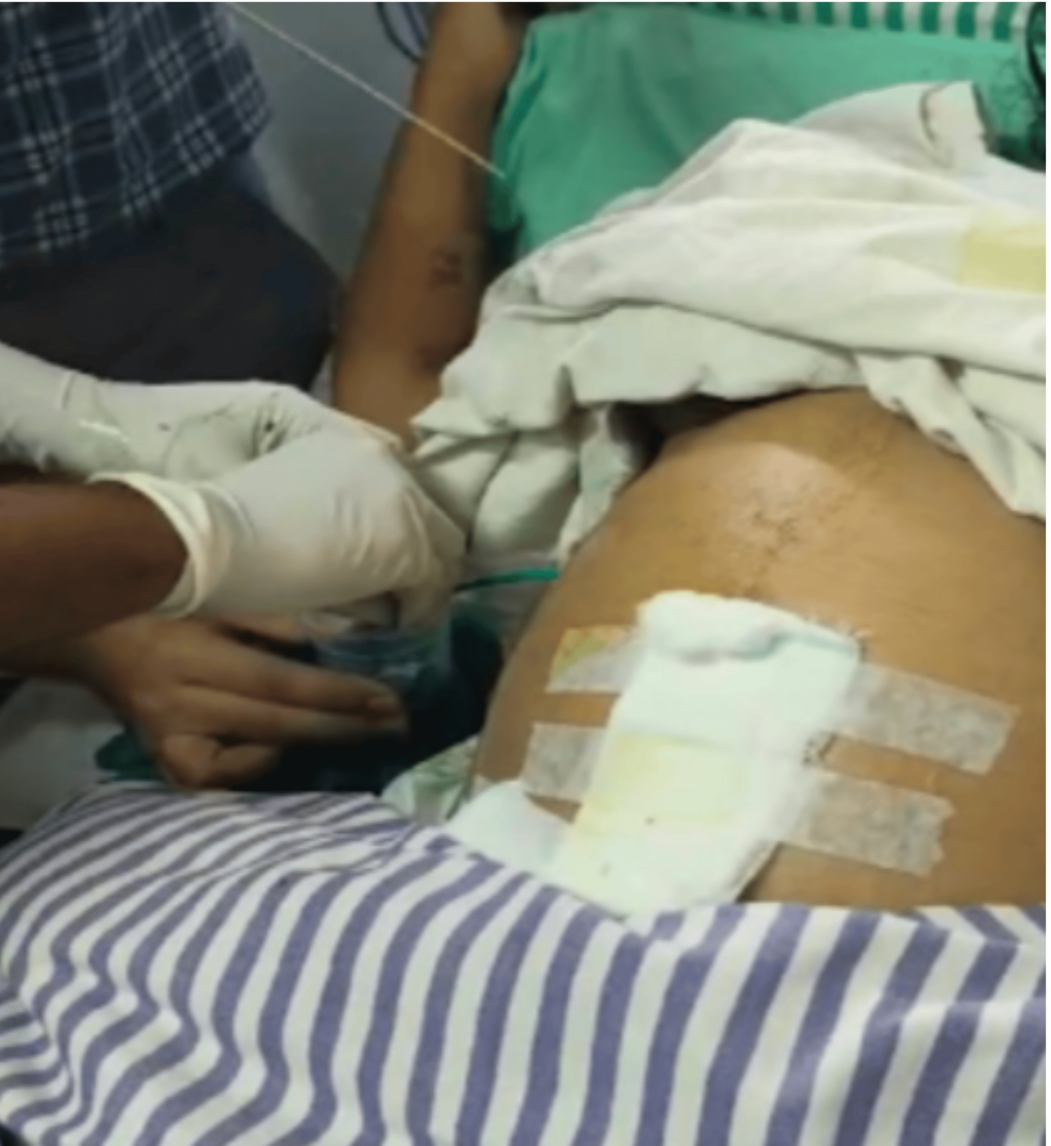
Ultrasound-guided percutaneous aspiration with pigtail placement - image 2 The image shows dressing over the midline and Pfannensteil sutures from a laparotomy performed outside at RMFK RMFK: Rural Medical Facility, Koppal
